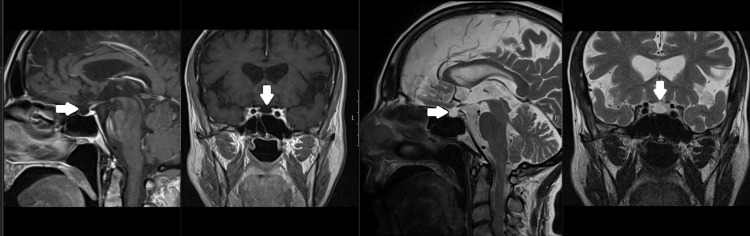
Cranial MRI images Cranial MRI of the patient showing an empty sella turcica (arrows) with pituitary tissue compressed against the sellar floor with lateral stalk deviation MRI: magnetic resonance imaging
